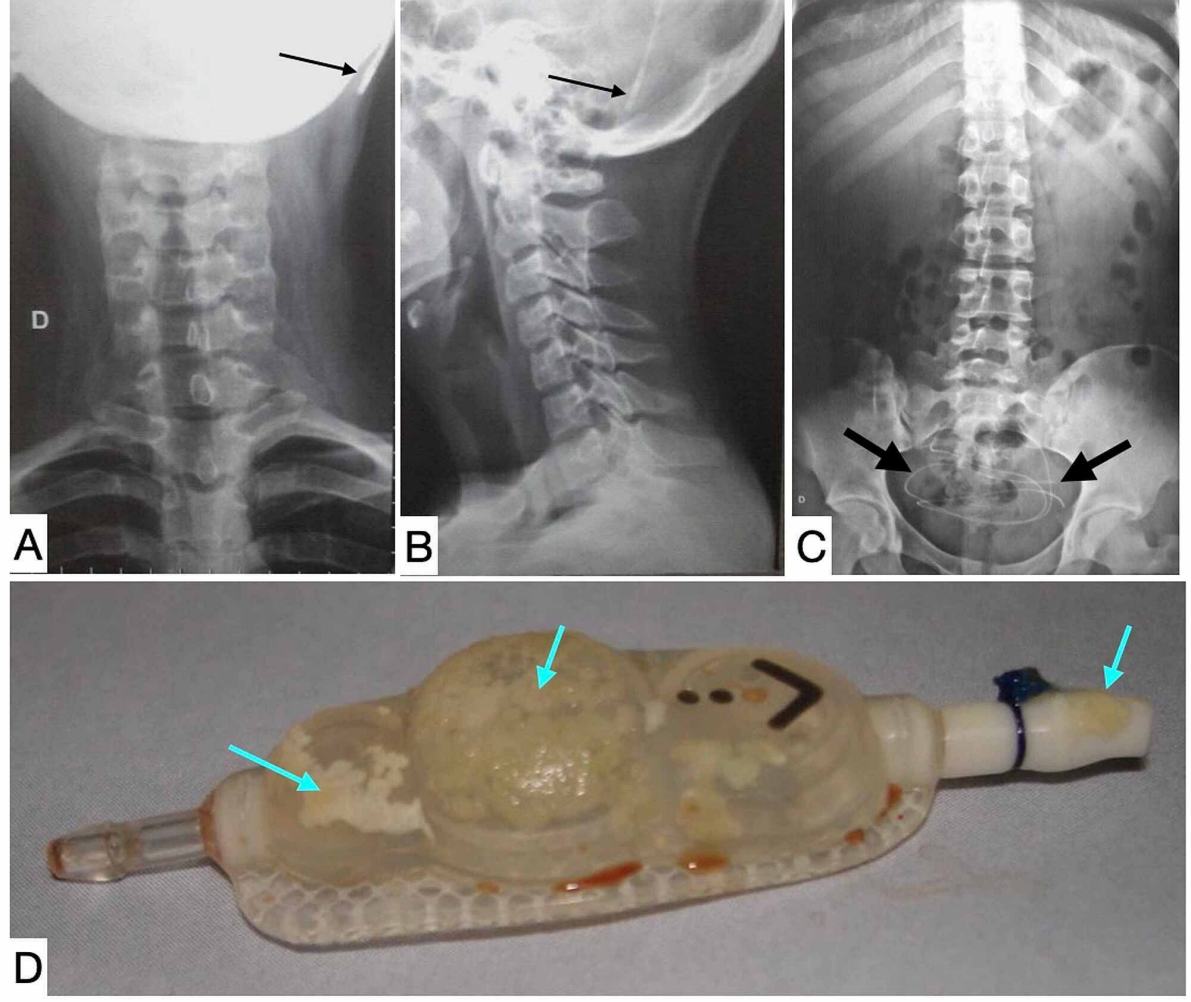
Shunt disconnection. The cervical X-ray displayed a shunt disconnection at the level of left retromastoid region (thin black arrow) in both projections anteroposterior (A) and lateral(B). The abdominal X-ray shows a complete migration of the distal catheter (thick black arrow) (C). The shunt mineralization as well of distal catheter was shown in this other patient (blue arrows) (D).
